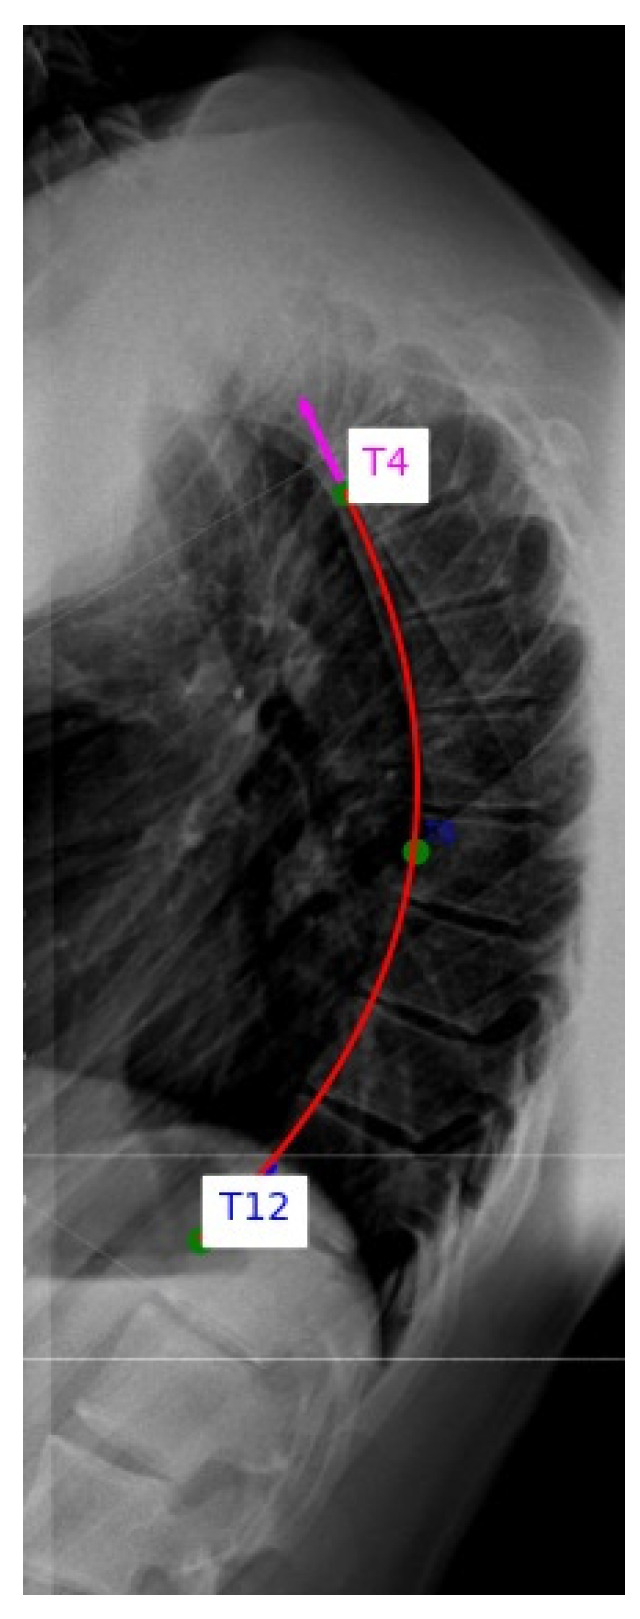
Custom Python tool for thoracic spine curvature analysis. The rater manually selects anterior wall midpoints of the triplet on lateral X-rays. The tool enables interactive landmark selection, automated spline and circle fitting and kyphotic angle calculation.
